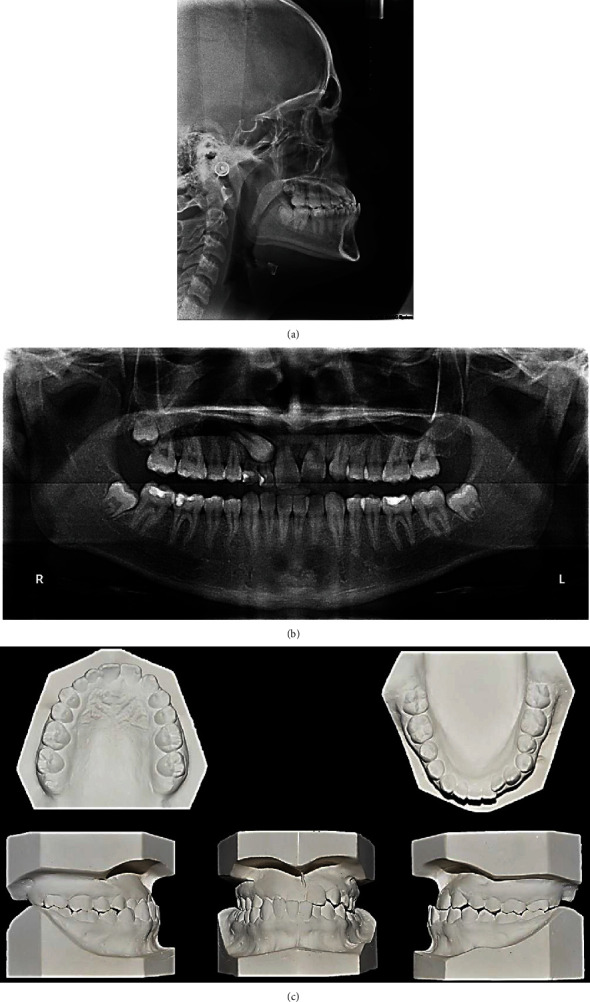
Pretreatment cephalometric radiograph, panoramic radiographs, and study models: (a) cephalometric radiograph, (b) panoramic radiographs, and (c) study models.
